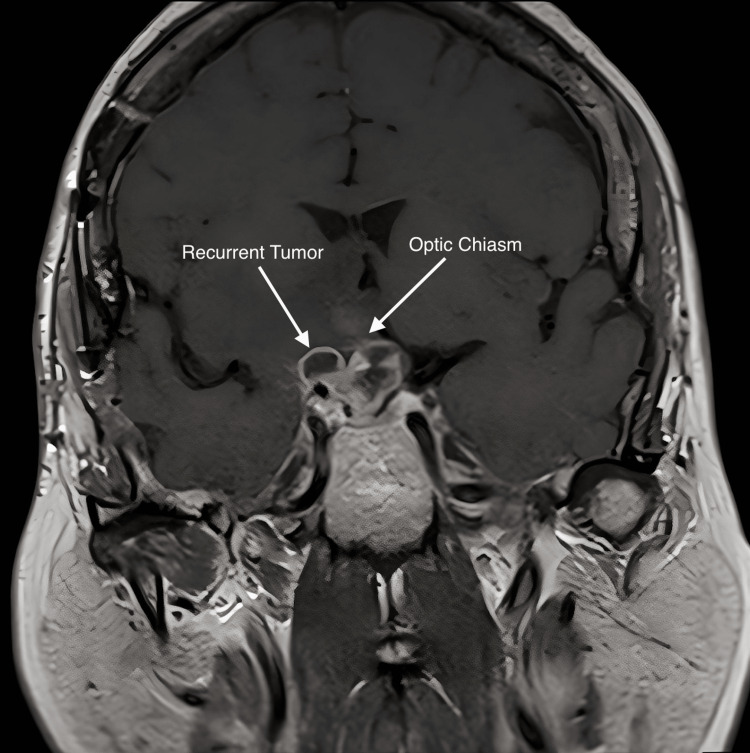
Mid-coronal MRI with contrast; this shows clinical progression and cystic enlargement of the tumor after 2160 cGy in 12 fractions
